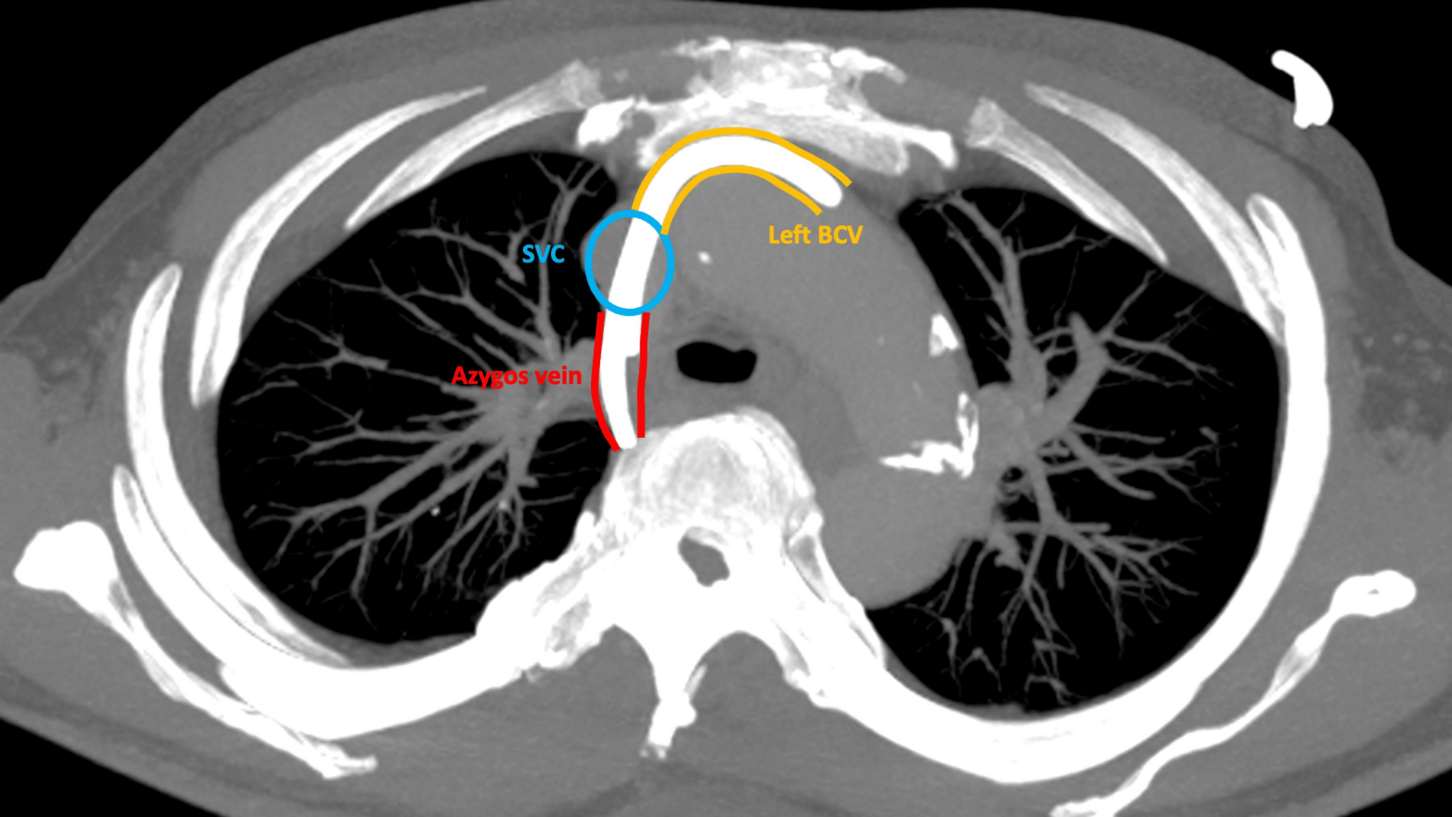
Contrast CT thorax of this case: MIP image in the axial plane The final portion of the left BCV (yellow vessel), where it meets the SVC (blue lumen), has an anterior-to-posterior trajectory. The catheter therefore preferentially entered the azygos ostium located on the posterior wall of the SVC, followed the azygos vein (red vessel), and traveled further posteriorly. MIP: maximum intensity projection, CT: computed tomography, BCV: brachiocephalic vein, SVC: malposition of central venous catheter
